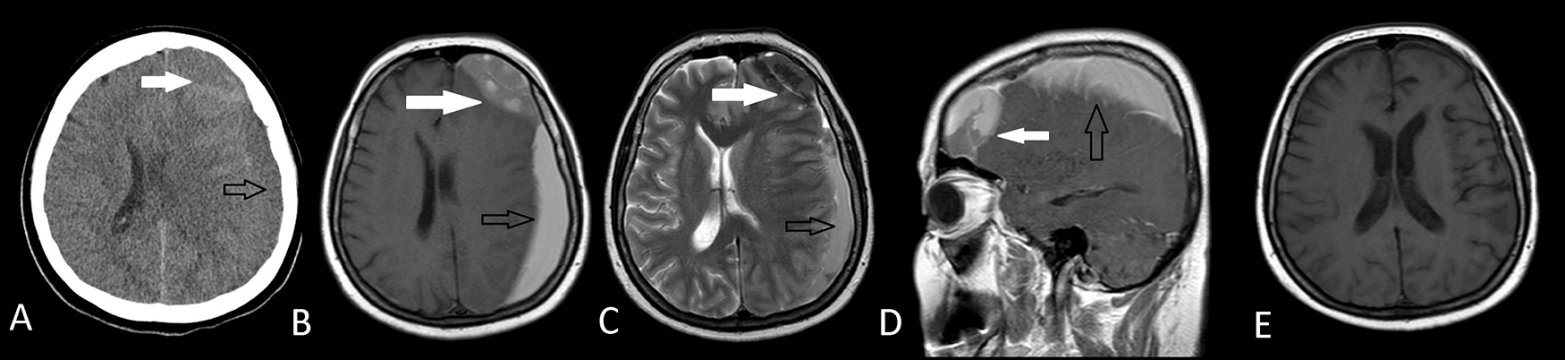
Preoperative axial CT scan (A) and axial T1W MRI (B) axial T2W MRI (C), sagittal MRI (D) images of an arachnoid cyst (white arrows) with ipsilateral subdural hematoma (black arrows) leading to a midline shift in Case 1. Arachnoid cyst had diagnosed before the onset of subdural hematoma and there was no history of a trauma. Postoperative axial T1W MRI (E) shows the total removal of both the arachnoid cyst and the subdural hematoma.
